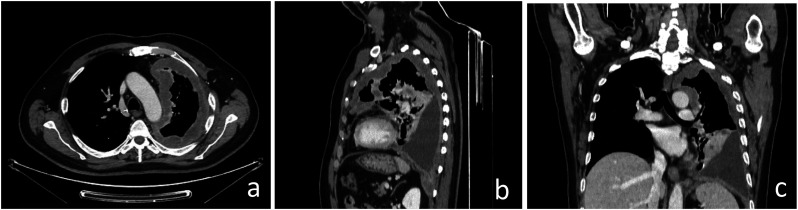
Example of malignant pleural mesothelioma studied with multidetector CT images in 3 planes with high spatial resolution (slice thickness of approximately 1-2 mm in the axial plane (a) and 2-3 mm in the sagittal and coronal planes (b and c).
